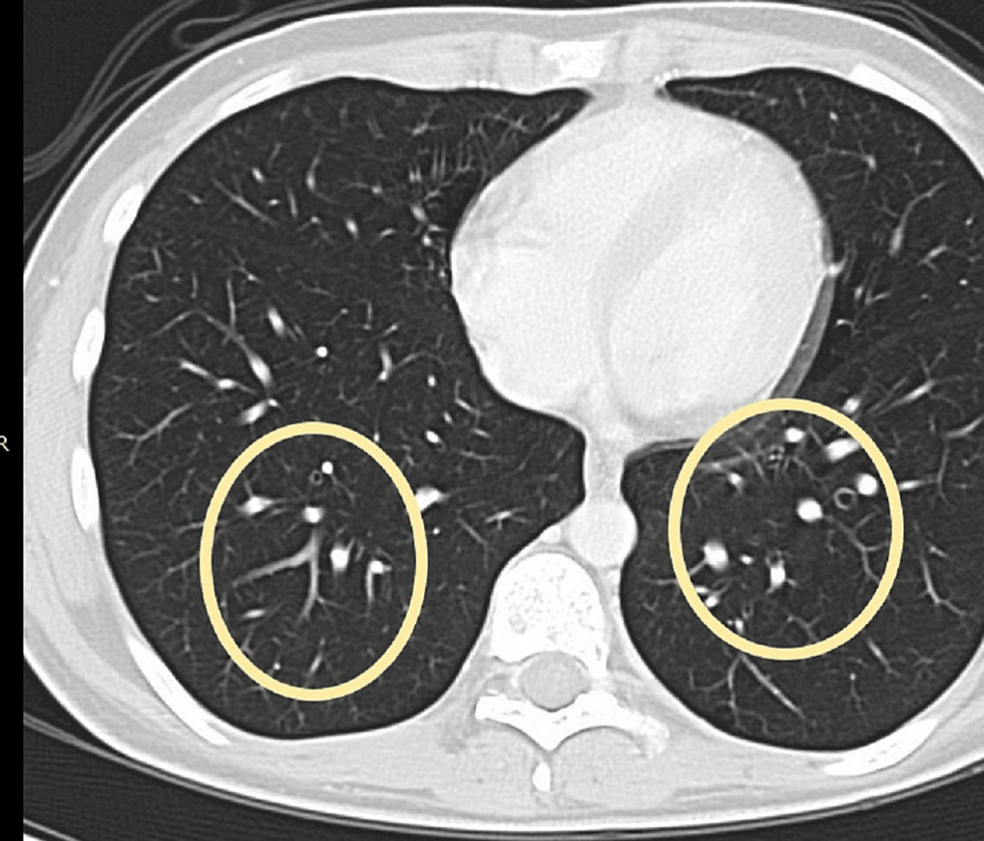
CT scan of the chest demonstrating multiple bilateral metastatic nodules (circles).
